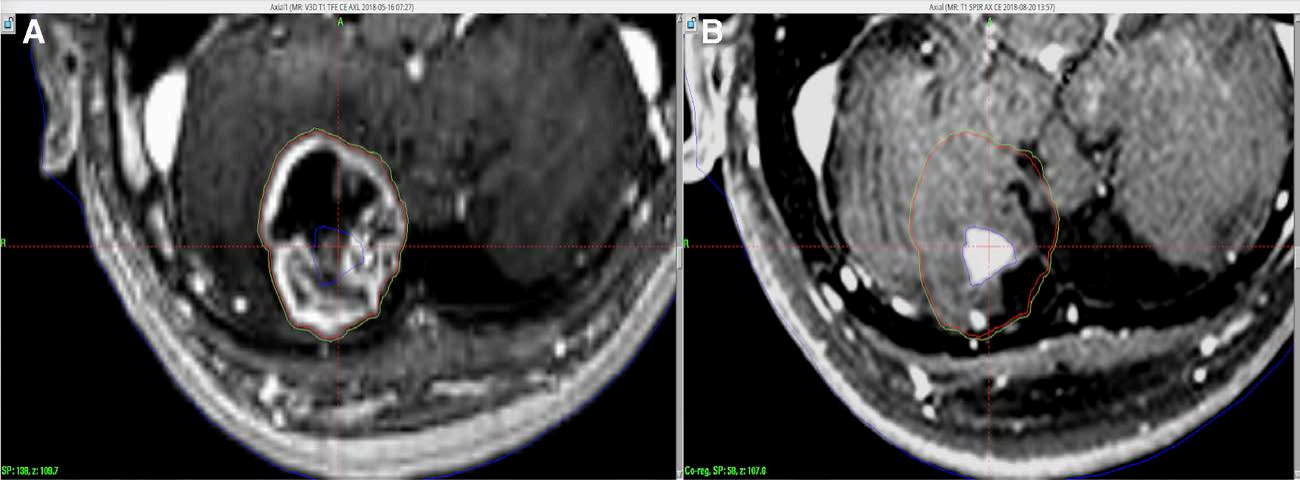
Follow-up MRI of case 1 after hypofractionated gamma knife radiosurgery. Pre (A) and post (B)-operative axial T1-weighted image. Contouring a tumor in red, margin in green. The tumor in blue was decreased at 3 months after hypofractionated gamma knife radiosurgery (follow-up MRI).
